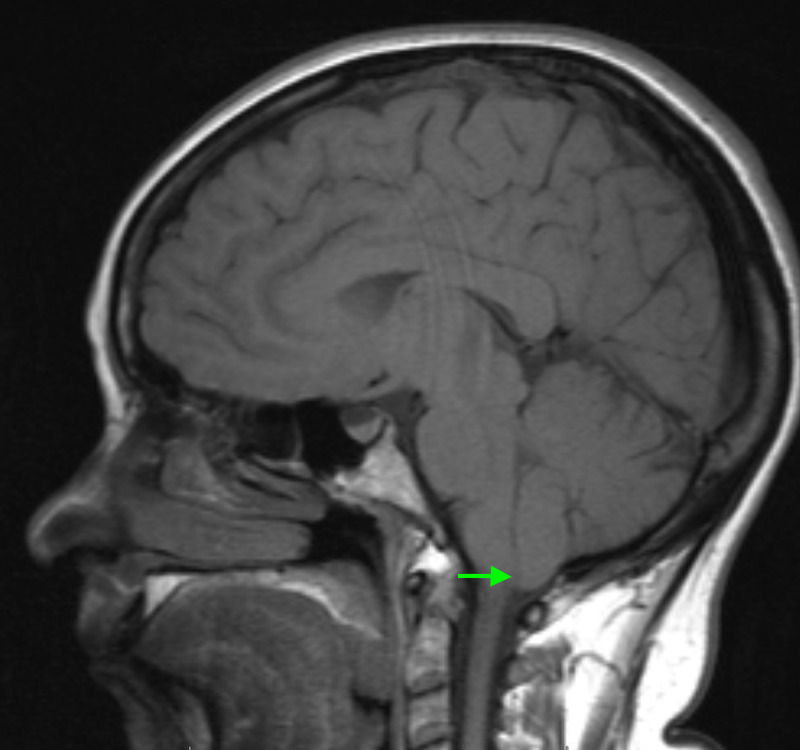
T1 sagittal MRI revealing a Chiari I malformation with approximately 5-6 mm of tonsillar herniation and no upper cervical syrinx.
